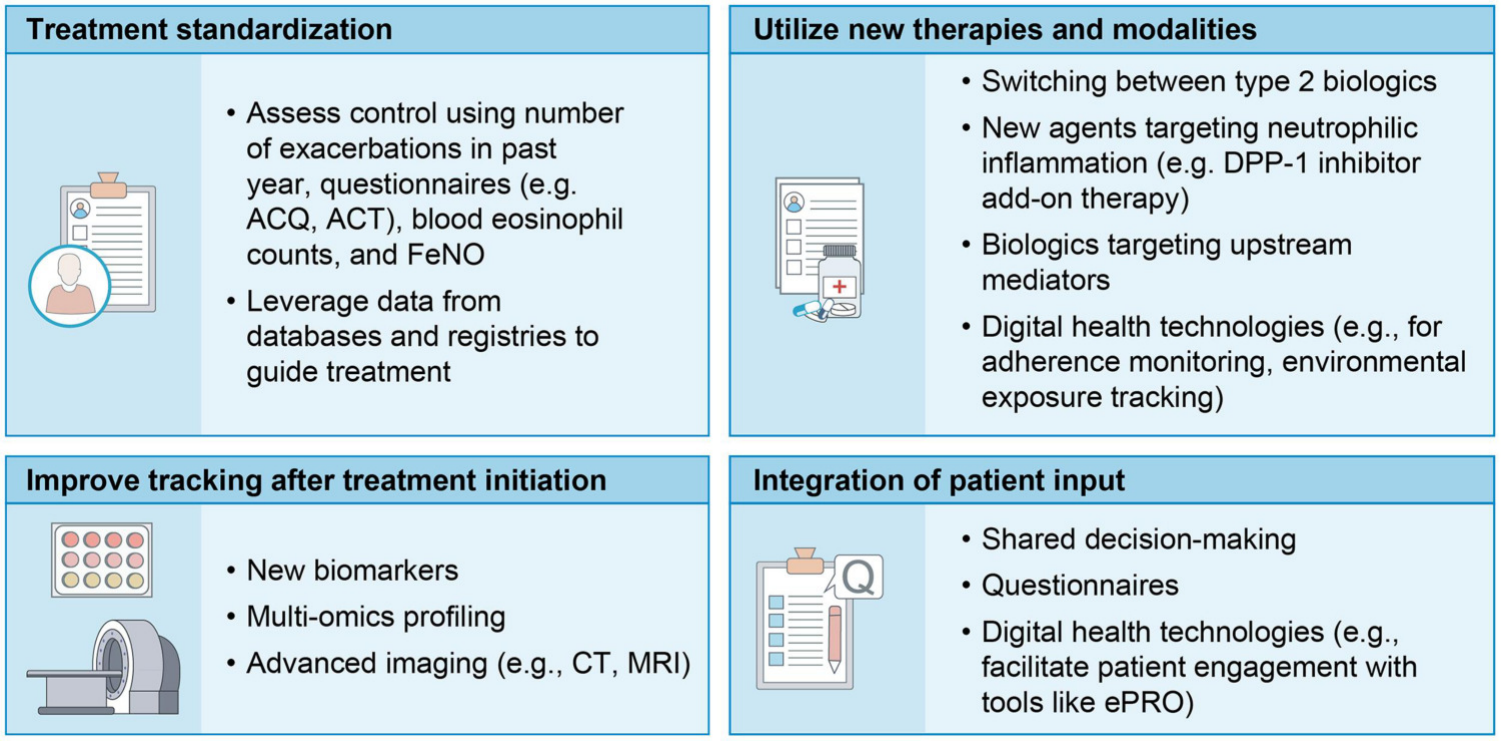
Looking to the future of uncontrolled severe asthma management. To further improve clinical decision-making and build on recent successes in severe asthma treatment, we should target four key areas of management. Treatment should be standardized based on clinical evidence to reduce heterogeneity, new therapeutic approaches should be incorporated, monitoring of asthma after treatment initiation should be improved using new biomarkers and imaging modalities, and patient input should be at the center during a shared decision-making process. ACQ, Asthma Control Questionnaire; ACT, Asthma Control Test; CT, computed tomography; DPP-1, dipeptidyl peptidase 1; FeNO, fractional exhaled nitric oxide; MRI, magnetic resonance imaging.
